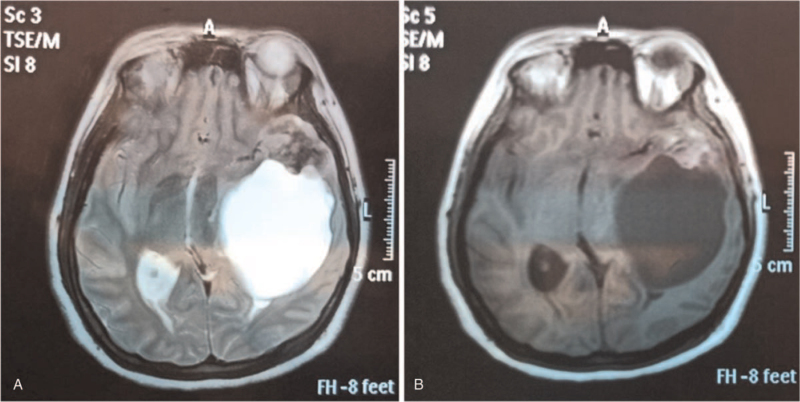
MRI showing a 7.5 × 5.0 × 5.0 cm sized cystic-solid mass in the in the left temporal. Cystic part of the tumor with a uniform long T1 and long T2 signalsss and the edge of is smooth. The solid part of the tumor with not regular edge shows inhomogeneous signal which is slight hypointense on T1-weigted images and slight hyperintense on T2-weighted images. There is no edema signal around the tumor. Tumor occupying effect is obvious, the left ventricle and lateral fissure pool are narrowly compressed and partially invisible. (Tumor occupying effect is obvious, compression of left ventricles and lateral fissure cistern narrowing or disappearance.). The midline structure shift to the right. There was no abnormality in the inner table of the adjacent skull. (A: Axial T1-weighted image. B: Axial T2-weighted image.) MRI = magnetic resonance imaging.
